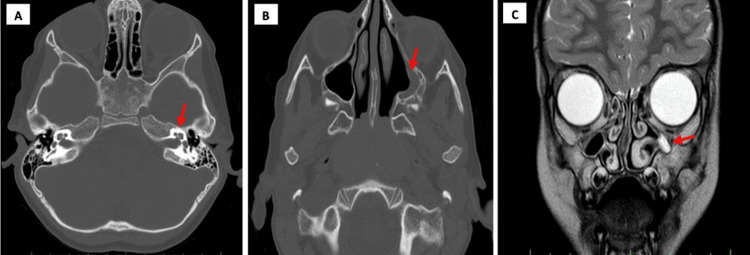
(A) Axial high-resolution computed tomography (HRCT) of the temporal bone. (B) Axial CT of paranasal sinuses. (C) Coronal magnetic resonance imaging (MRI) (A) Axial high-resolution computed tomography (HRCT) of the temporal bone showing normal bony inner ears and petrous bone on both sides. (B) Axial CT of paranasal sinuses showing normal right maxillary sinus with retraction and shrinking of the left maxillary sinus with rudimentary mucosa in the lateral wall. (C) Coronal magnetic resonance imaging (MRI) showing fully opacified left maxillary sinus with thickening and enhancement of the mucosa.
